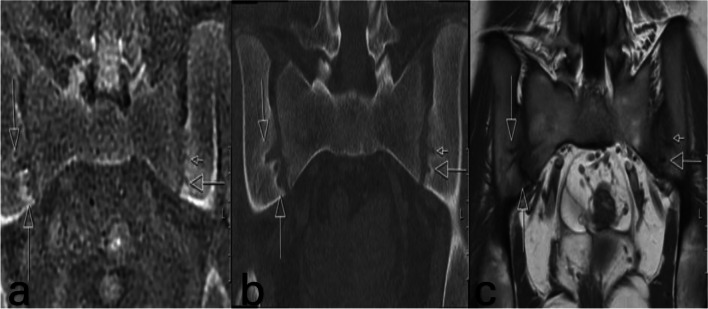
A 16-year-old girl with sacroiliitis. a Coronal ZTE-MRI shows cortical erosion areas (*arrow*). b Coronal low-dose CT image shows cortical erosion areas (*arrow*). c Coronal T1-weighted image indicates areas of cortical erosion (*arrow*), but these appear smaller. *CT*, computed tomography; *ZTE-MRI*, zero–time echo magnetic resonance imaging
